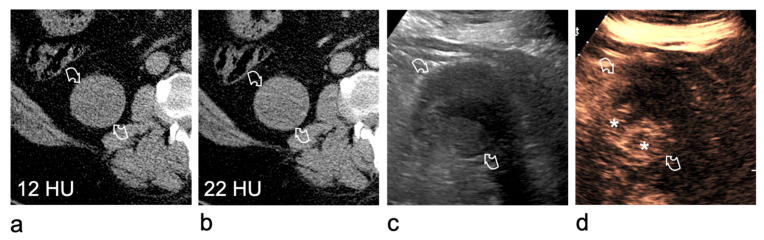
Utility of CEUS for characterising a right renal lesion (curved arrows) with equivocal enhancement on CT. (a) On the unenhanced scan, the right renal lesion measures 12 HU. (b) In the nephrographic phase after contrast administration, it shows equivocal enhancement (22 HU). (c) B-mode US remains non-diagnostic because the lesion is inhomogeneously echogenic. (d) CEUS unequivocally demonstrates an enhancing mural vegetation (asterisks), consistent with a Bosniak category IV lesion. Surgery revealed a low-grade clear cell renal cell carcinoma.
